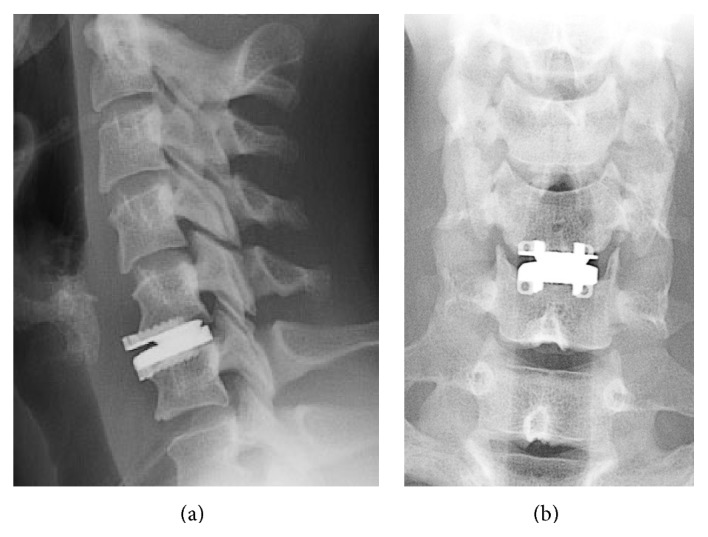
Postoperative lateral (a) and AP (b) radiographs demonstrating that the implant is well positioned on the AP (anteroposterior) view and slightly prominent on the lateral view.
